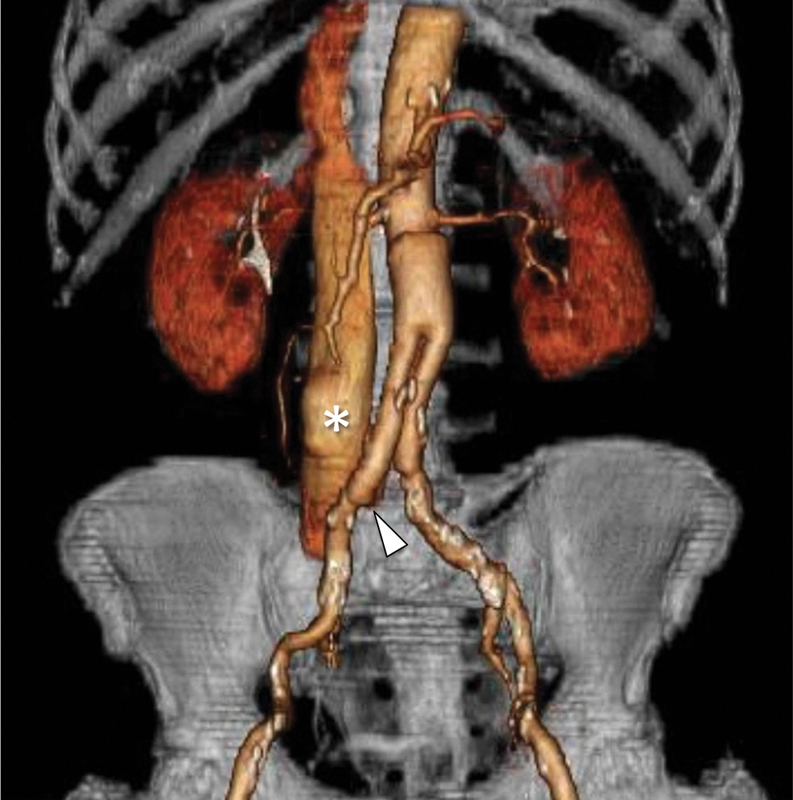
Abdominal contrast medium-enhanced multidetector computed tomography coronal volume rendering technique reconstruction that shows, at the level of the right iliac anastomosis, the presence of fissured pseudoaneurysm (arrowhead) in communication with the proximal third of the inferior vena cava (*).
